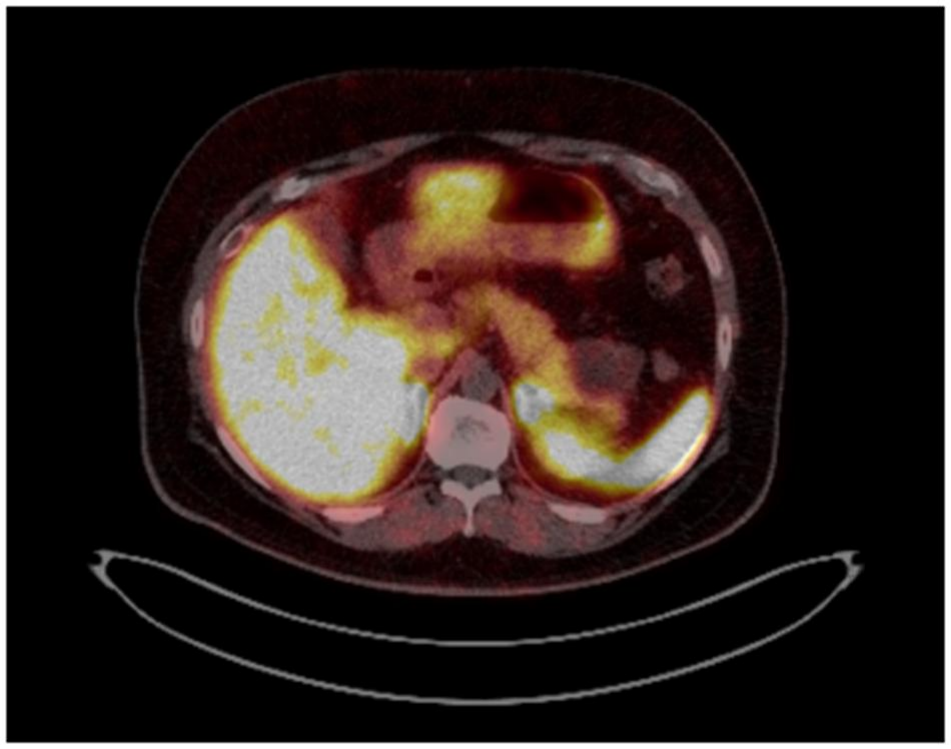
Gallium PET-CT performed showed no somatostatin receptor avidity in the pancreatic lesion.
